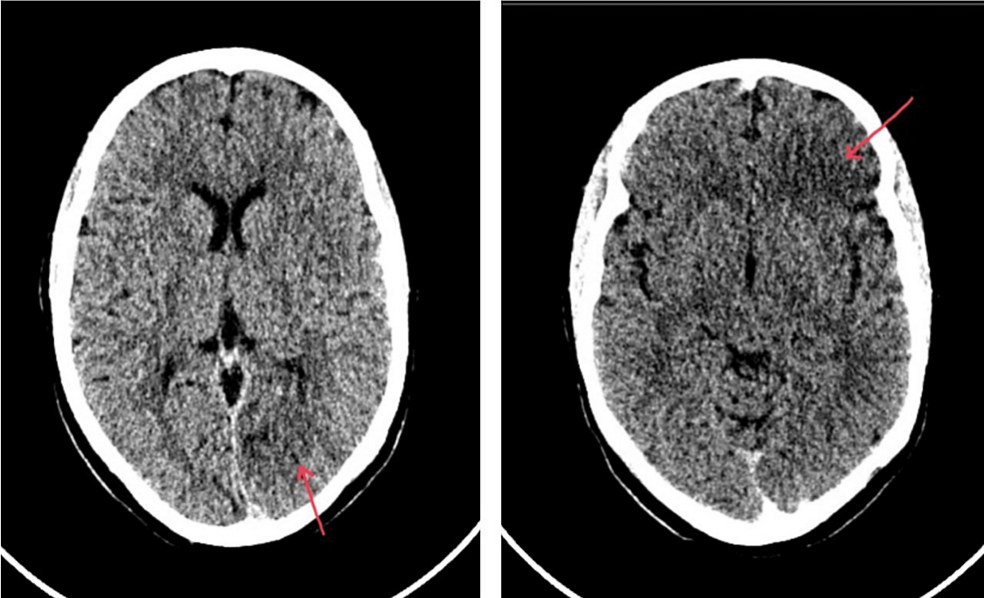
Computed tomography of the brain without contrast shows distal infarctions in the frontal and occipital regions.
